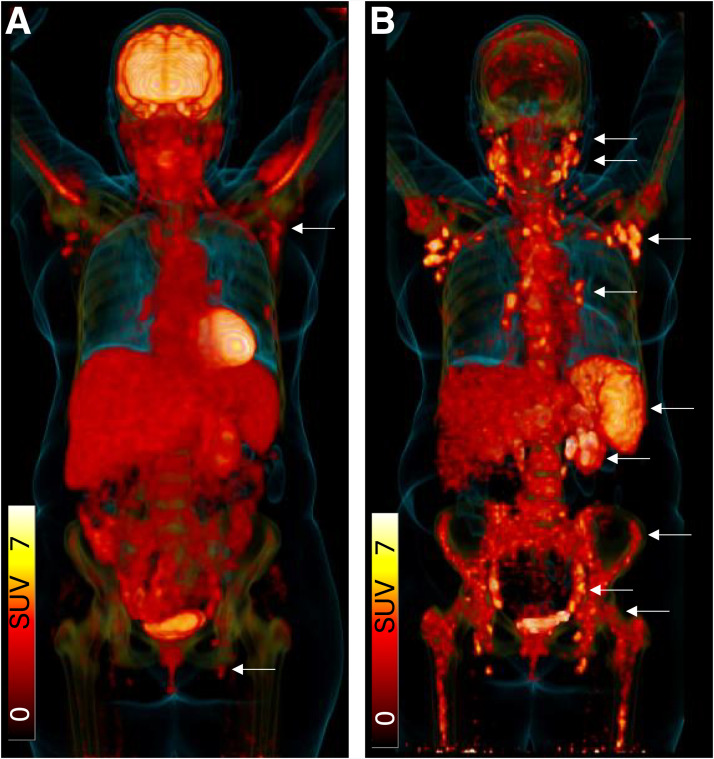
In hematologic neoplasms, CXCR4-directed PET can be more sensitive than [^18^F]FDG PET, particularly in marginal zone lymphoma. PET/CT scans of a 70-y-old woman with initial diagnosis of marginal zone lymphoma are shown, performed within 2 d of each other. (A) [^18^F]FDG PET/CT (3-dimensional view) shows moderately increased tracer uptake in axillary and inguinal lymph nodes (arrows). (B) [^68^Ga]Ga-Pentixafor PET/CT (3-dimensional view) shows additional lesions in cervical, mediastinal, parahepatic, and pelvic lymph nodes as well as bone marrow compartment and spleen, indicating advanced disease.
